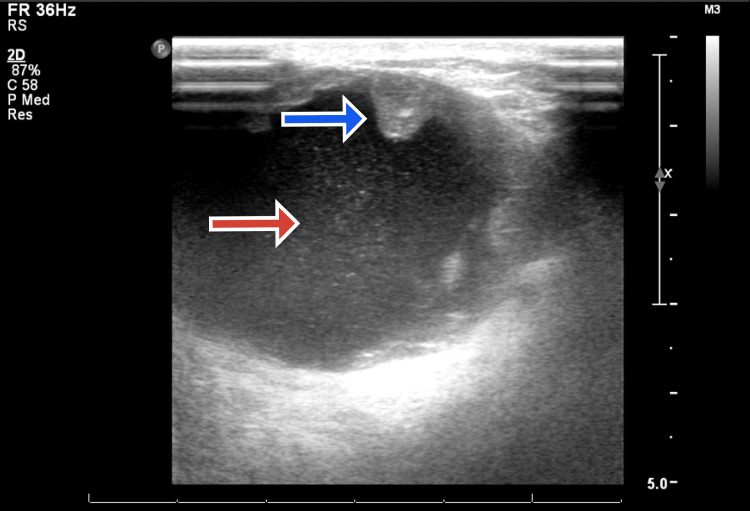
Ultrasound showed a hypoechoic area with discrete areas of calcifications with a rim of peripheral enhancement. Blue arrow shows the solid component in a non-dependent position suspended in the cyst shown by the red arrow.
